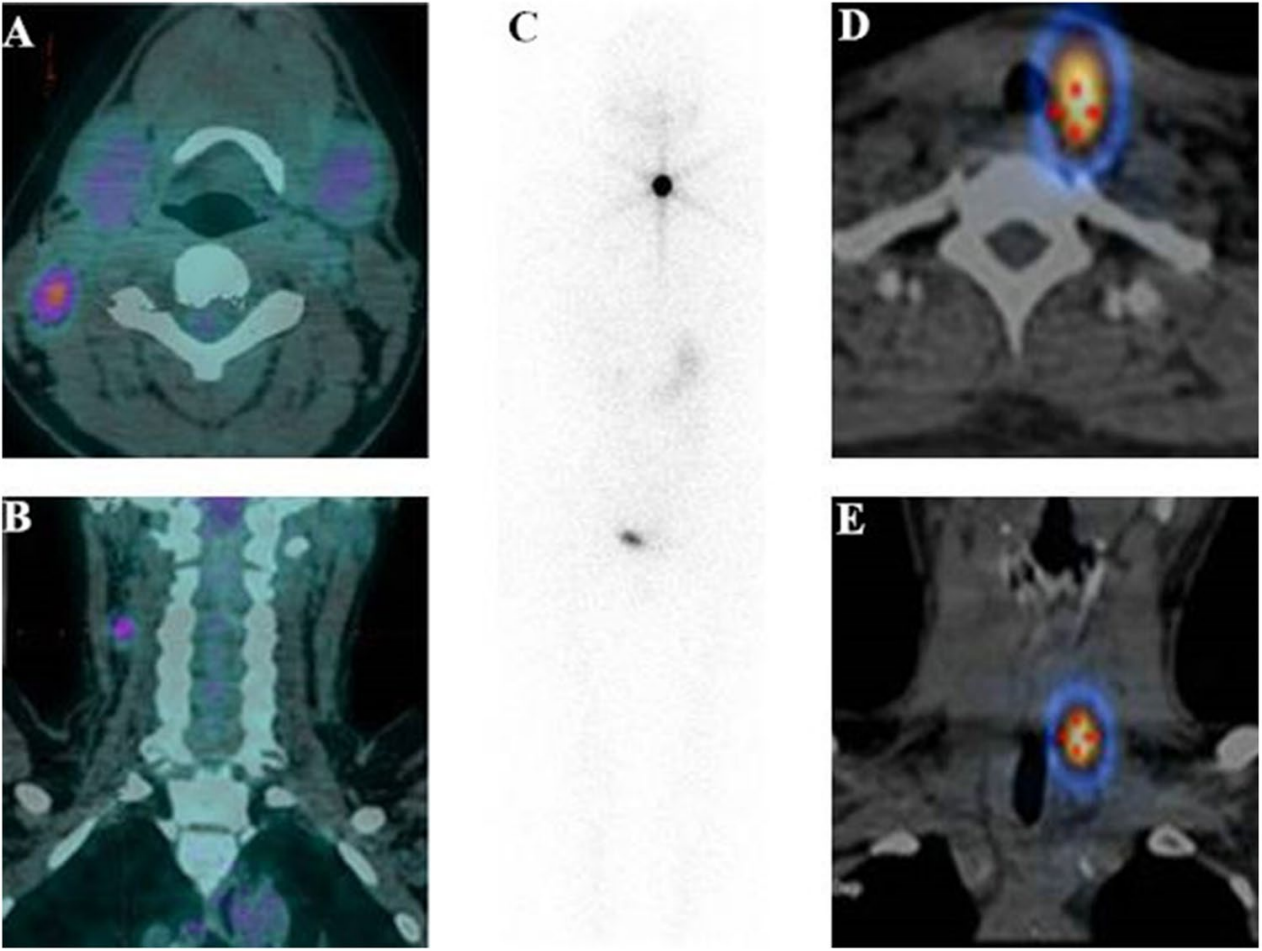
^18^F-FDG-avid papillary thyroid carcinoma (PTC) metastatic lymph nodes non-avid for ^131^I. A 32-y-old female with PTC presented with suspicious metastatic disease 2 months after total thyroidectomy with elevated stimulated serum throglobulin (ssTg) of 78 ng/mL and thyroid-stimulating hormone (TSH) of 102.4 mU/L. Transaxial (A) and coronal (B) fusion images of ^18^F-FDG PET/CT before the administration of 5.55 MBq (150 mCi) of ^131^I showed obviously radiotracer-avid lymph nodes (LN) (SUVmax = 4.6) in the neck. The ^131^I whole body scan (C) and transaxial (D) and coronal (E) fusion images of ^131^I SPECT/CT of the neck revealed no ^131^I accumulation in the lymph node (not shown) but ^131^I uptake in the thyroid remnant.
